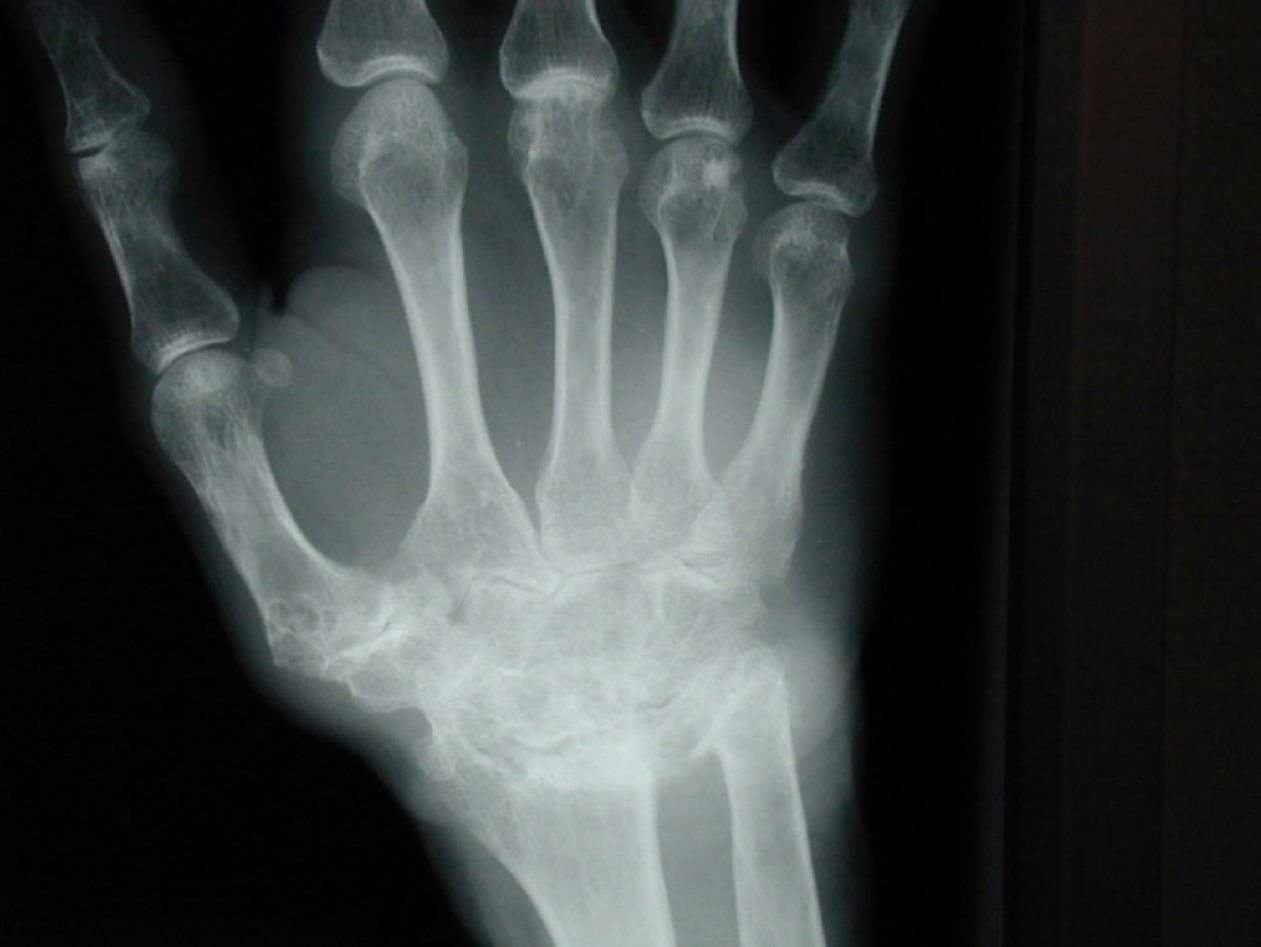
Case 3– Right preoperative X-ray a.p. view
